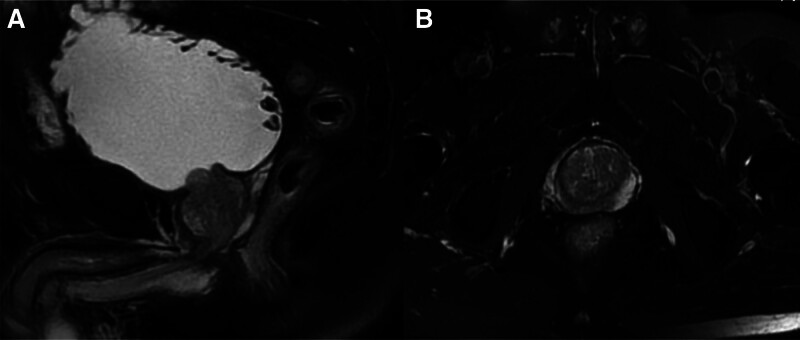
The volume of prostate increased, the boundary of prostate was clear, and the upper edge of the hyperplastic prostate protruded into the bladder (A). The prostate volume of the central zones and the transitional zones increased, the T2WI signal of prostate was moderate, mainly with stromal hyperplasia of prostate, peripheral zones of prostate was compressed and thinned, the capsule was intact (B). T2W1 = T2 weighted image MRI.
